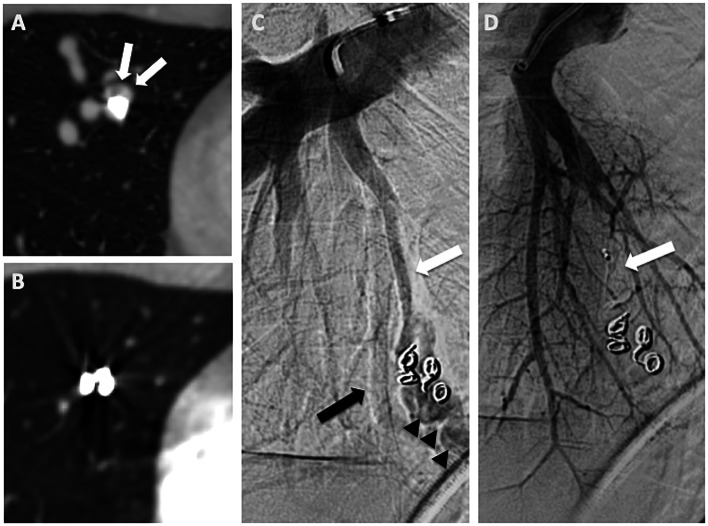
Right middle lobe pulmonary AVM in a 57-year-old female patient with hereditary haemorrhagic telangiectasia which was treated 5 years earlier with coil embolisation. Axial follow-up CT slice (A) showing recanalisation after coil embolisation (white arrows). Axial CT slice 6 months after treatment (B) of recanalised PAVM showing successful occlusion with no persistence of PAVM. Pulmonary angiogram of recanalised PAVM (C) showing feeding artery (white arrow), venous sac (black arrowheads) and draining vein (black arrow) filling with contrast despite previous coil embolisation. Pulmonary angiogram (D) showing successful occlusion of PAVM with Amplatzer vascular plug (white arrow) and no filling of venous sac
